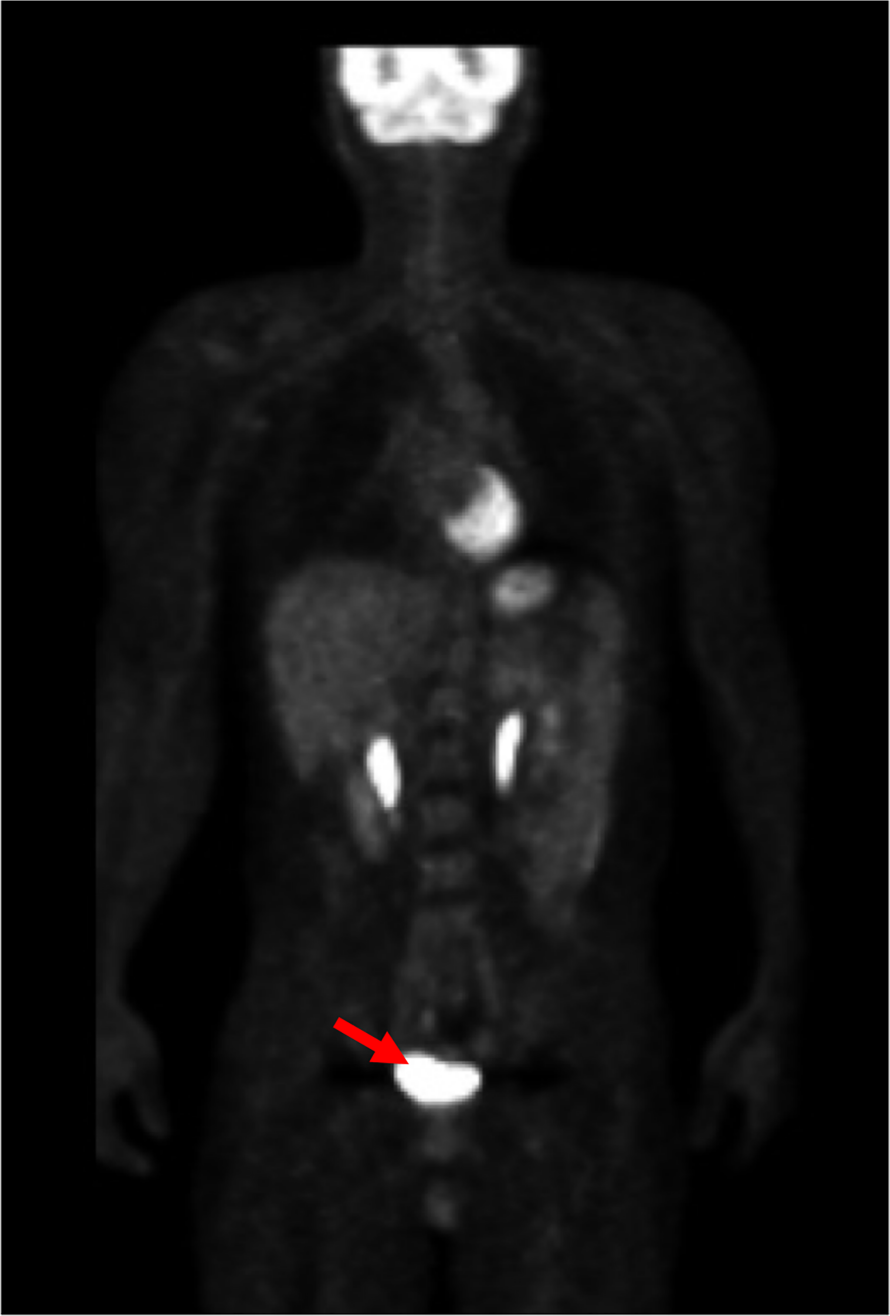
Example of normal accumulation (bladder). The SUV of 65.4 was converted into Z-score of 1.84, because the mean and SD at the location (an arrow) were 19.6 and 24.9, respectively.
